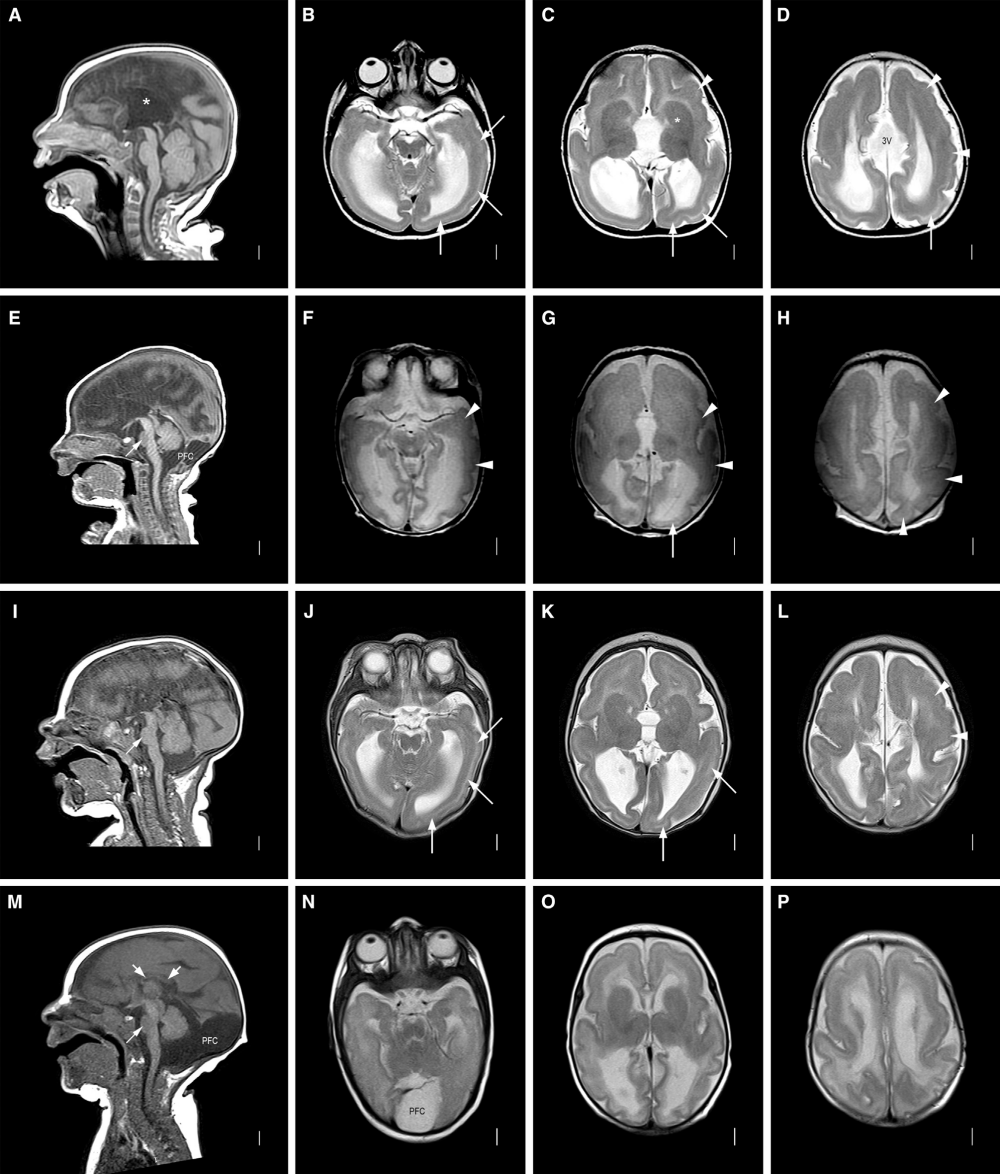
Neuroradiology of affected individuals Neuroimaging in four individuals with the *CAMSAP1*-related neuronal migration disorder (for further imaging see Figure S2): row 1 (A-D) is family 1, V:1 aged 7 months; row 2 (E-H) is family 2, II:1 aged 2 days; row 3 (I-L) is family 3, II:1 aged 3 months; row 4 (M-P) is family 4, II:1 aged 3 months. T1-weighted midline sagittal images show absent (∗ in A, also E and I) or short and thin (short white arrows in M) corpus callosum and small base of the pons (thin white arrow in E, I, and M). Enlarged posterior fossa or “mega cisterna magna” (PFC in E and M) was seen in 2/4 subjects. T2-weighted axial images show posterior-more-severe-than-anterior gradient with areas of agyria or severe pachygyria with prominent cell sparse zones and reduced thickness of the cerebral mantle/wall in posterior regions (white arrows in B–D, G, J, and K) and areas of less severe pachygyria with thicker cerebral mantle/wall in anterior regions (white arrowheads in C, D, F–H, and L). The gradient in family 4, II:1 (N–P) was less clear with lower resolution images. The boundaries of the basal ganglia and thalami were difficult to see, and the internal capsules are not seen (^∗^ in C, also in G, K, and O). The third ventricle was enlarged in all and dramatically enlarged into a midline interhemispheric cyst in family 1, V:1 (3V in D).
